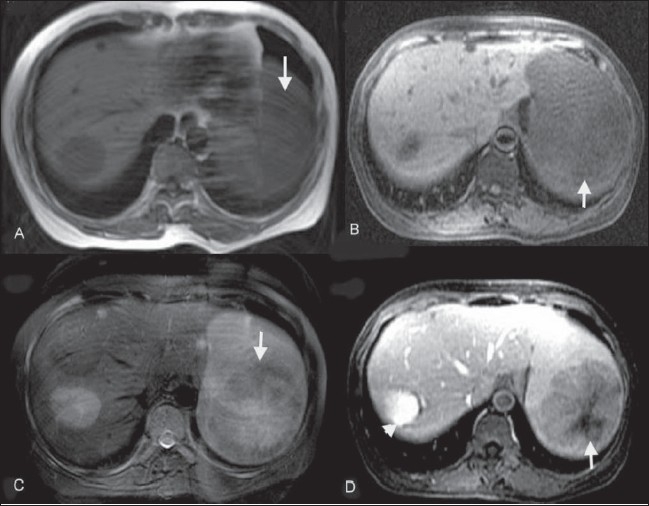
LCA of spleen. Case 1. MRI. Axial spin-echo T1W (A), axial gradient-echo T1W (B) and axial fast spin-echo T2W (C) images show a hypointense splenic mass (arrows). A contrast-enhanced image (D) shows heterogeneous enhancement (arrow). An incidental hemangioma is seen in the right lobe of the liver (arrowhead in D)
